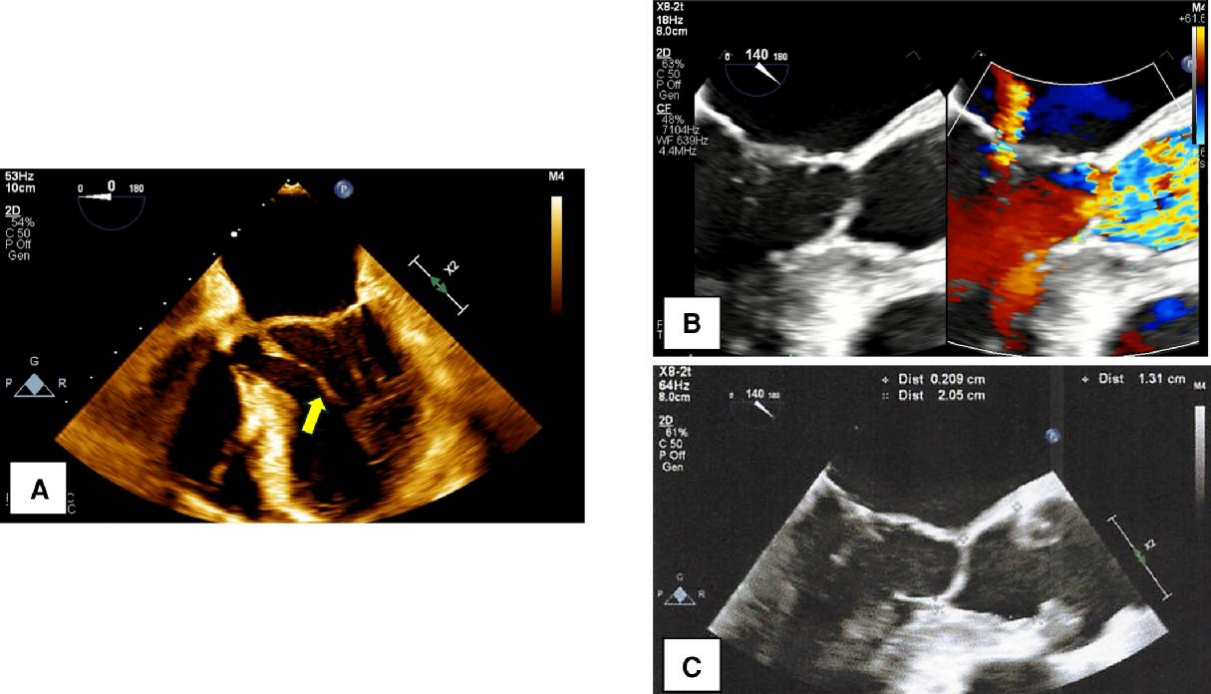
Transoesophageal echocardiogram findings. (*A*) Sepia-toned four chamber view. Abnormal chordae were observed attaching to the basal anterior mitral leaflet and the posterior papillary muscle. (*B*, *C*) Zoomed image in left ventricle outflow tract of left ventricle and aorta long axis view. The accelerated blood flow in the LVOT with mosaic coloured Doppler was observed. The narrowest point was approximately 20 mm below the aortic valve.
